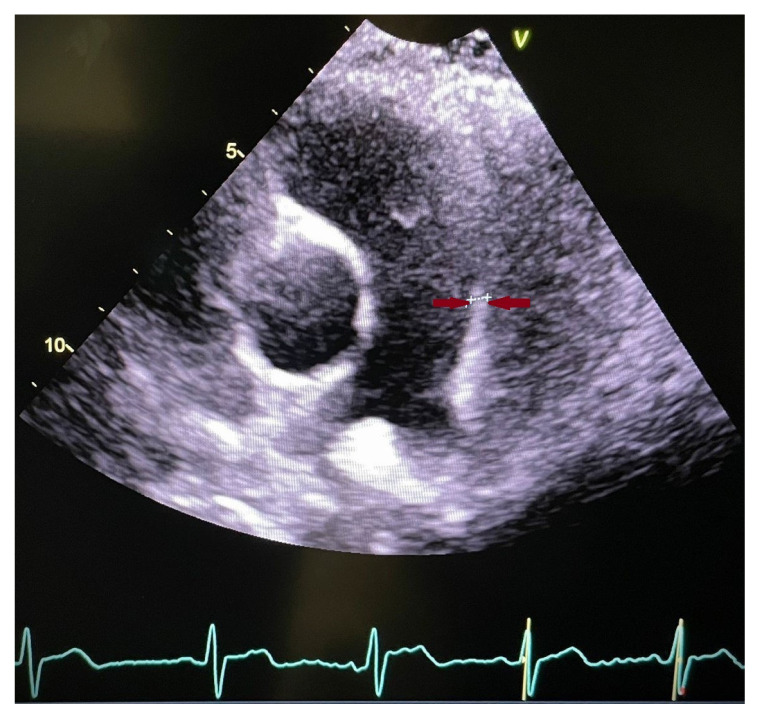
Echocardiographic measurement of pulmonary artery vessel wall thickness.
